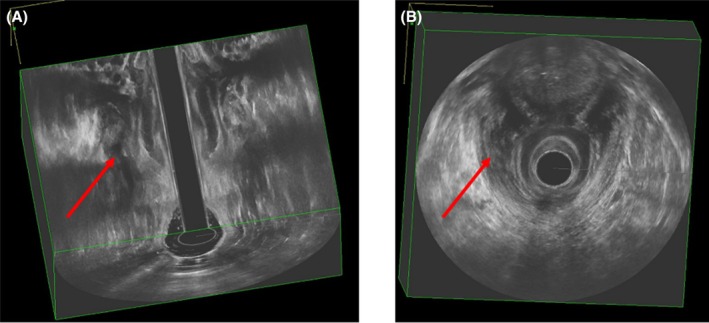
Endoanal ultrasound demonstrating closure of the secondary tract shown in Figure 4. (A) Coronal plane. (B) Axial (transverse) slice. Red arrows indicate the site of the previously outlined secondary tract; interval imaging shows no discrete tract. The central artefact corresponds to the probe footprint.
